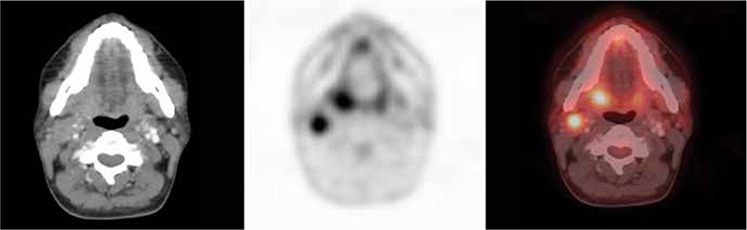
A 39-year-old-female patient with a diagnosis of metastatic squamous cell carcinoma on cervical node biopsy. Axial positron emission tomography-computed tomography and fusion images display hypermetabolic right level II cervical node (SUVmax: 5.9) and right tonsillar soft tissue mass (SUVmax: 6.5) which turned out to be tonsillar squamous cell carcinoma
